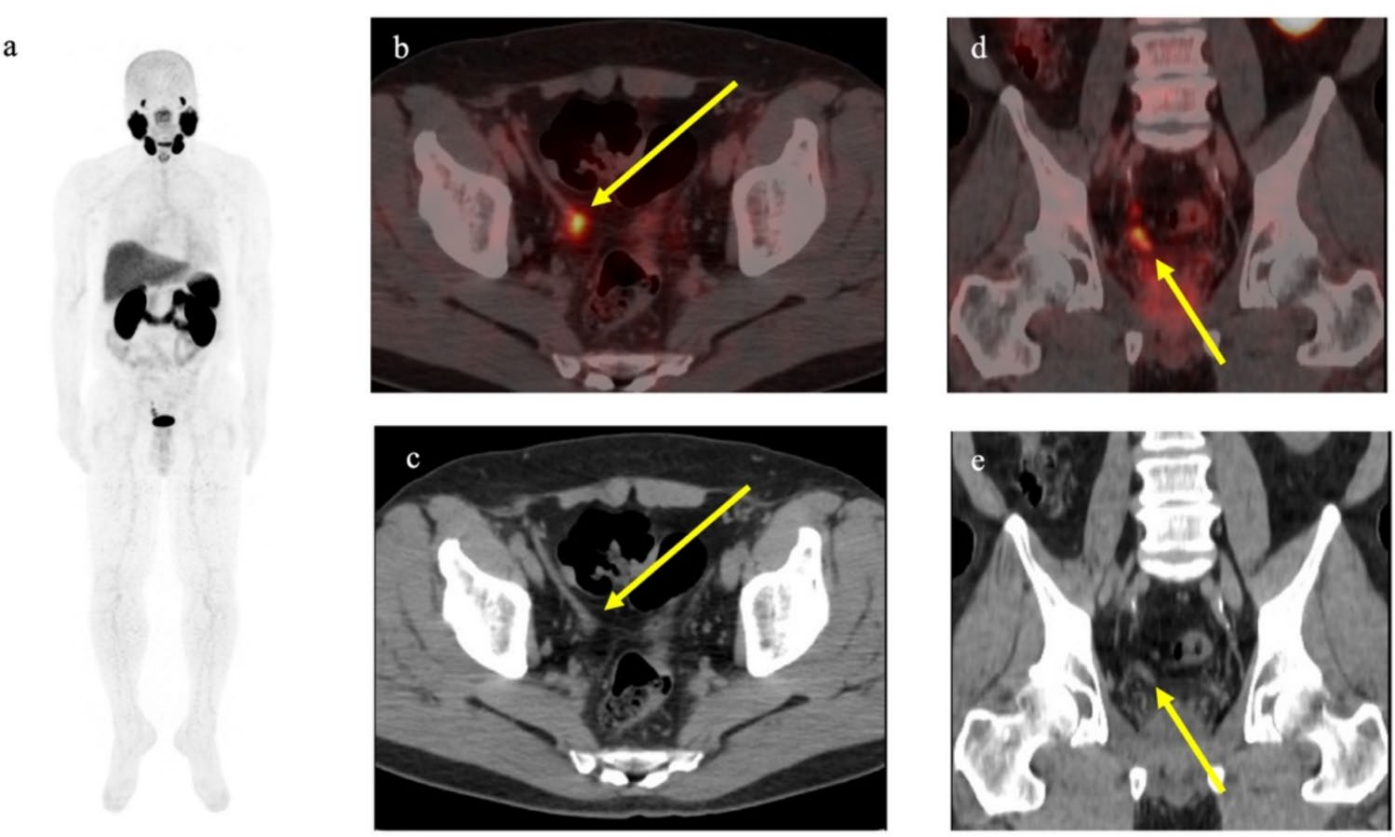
PET/CT using ^68^ Ga-PSMA-11 in a 71-year-old man with biochemical recurrence after radiation therapy (cT3aN0, Gleason score 4 + 5). PSA level increased to 8.20 ng/ml. Focal uptake was observed from the right seminal vesicle to the vas deferens, with no other significant uptake apparent, suggesting metastasis. Combined androgen blockade therapy was administered and the PSA level decreased to 0.04 ng/ml. a Maximum intensity projection image. b Transaxial PET/CT fusion image. c Transaxial CT. d Coronal PET/CT fusion image. e Coronal CT
